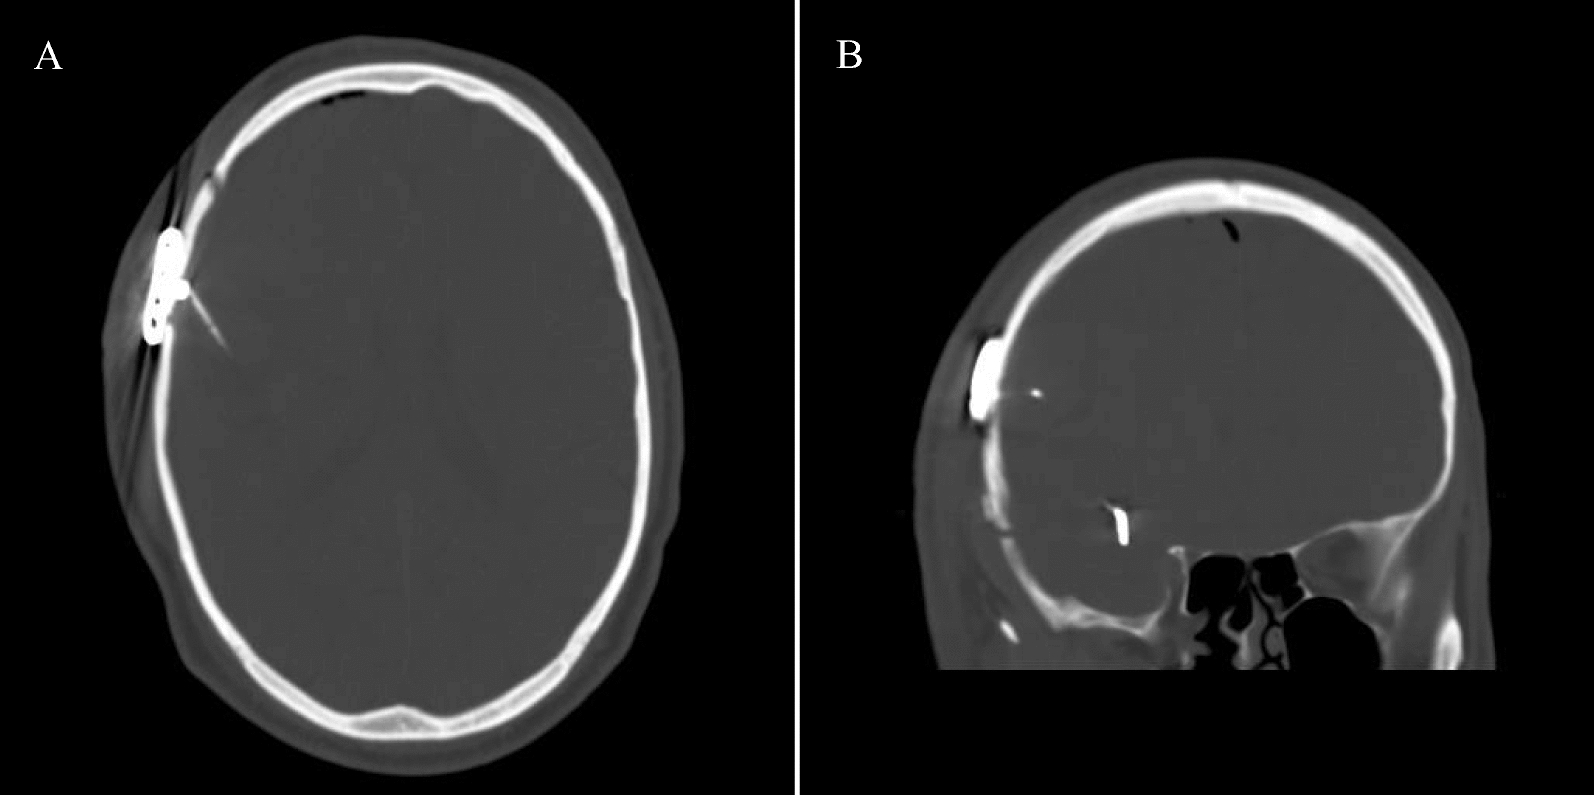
CT scan of telemetric ICP sensor. The figure displays coronal and axial CT scans of a patient, highlighting the implanted telemetric sensor. Routine postoperative CT scans following the clipping of unruptured aneurysms are not standard practice within our neurosurgical department and are reserved for cases where complications are suspected. Consequently, the CT scan depicted in this figure originates from a patient who was excluded due to postoperative bleeding
